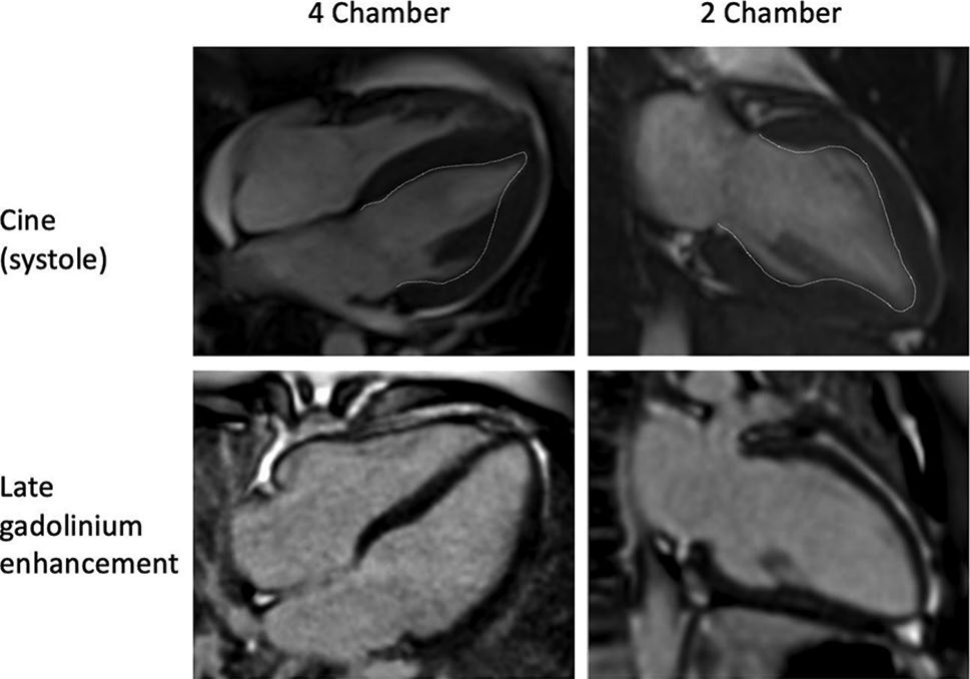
Cardiac magnetic resonance imaging demonstrating mid ventricular hypokinesis (with the endocardial border being highlighted [top panel]), and no late gadolinium enhancement (bottom panel)
